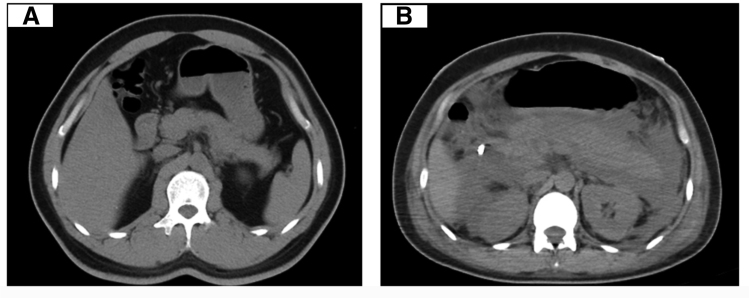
CT characteristics in MAP and SAP: (A) MAP, (B) SAP. CT = computerized tomography, MAP = mild acute pancreatitis, SAP = severe acute pancreatitis.
